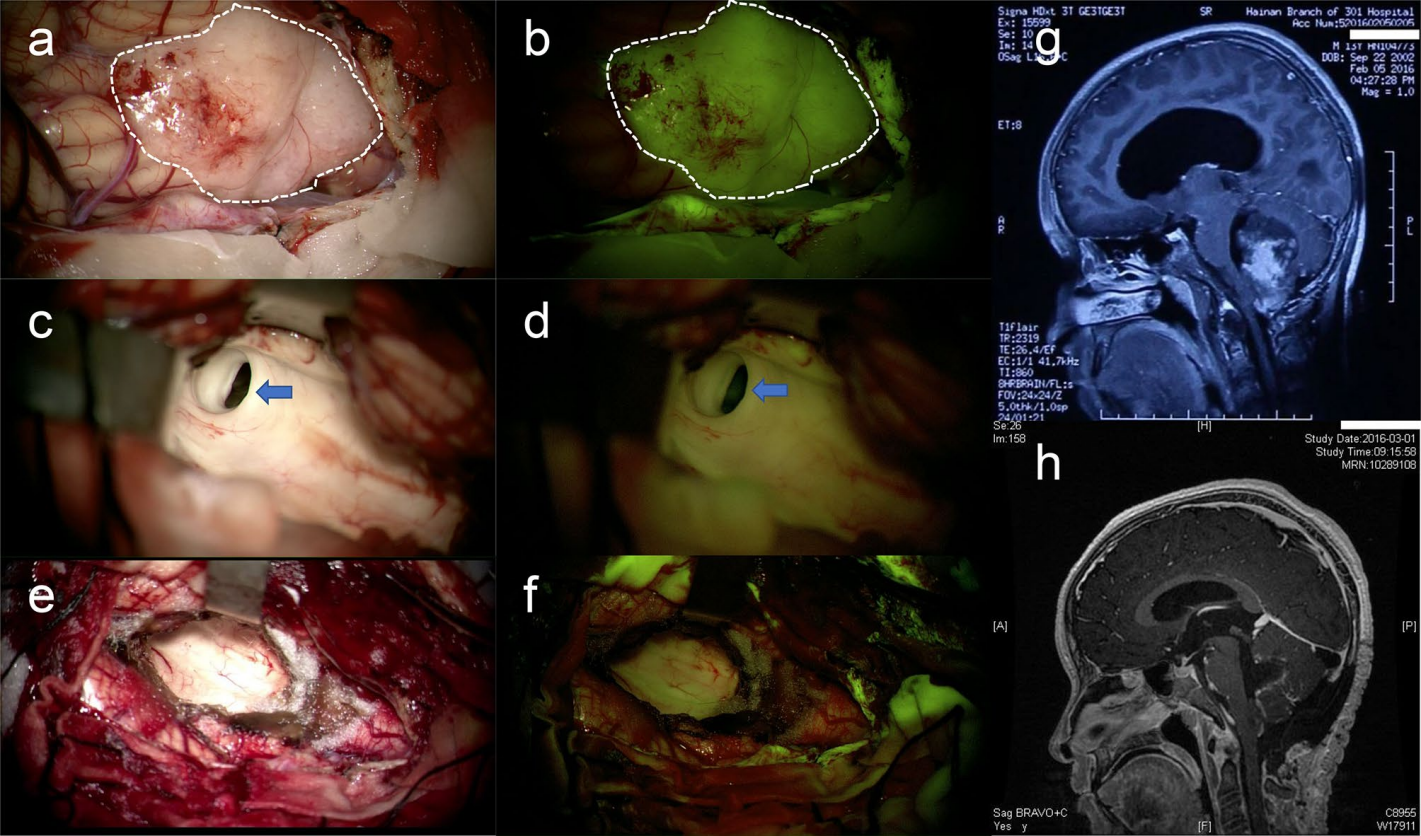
An 8-year-old boy underwent fluorescein sodium guided surgery for medulloblastoma of the cerebellar vermis. a The tumor (showed the white dashed frame) herniated from the cerebellomedullary fissure under the white light and (b) the yellow light. When the tumor was removed, the opening of the midbrain aqueduct (c, the white light mode; d, the yellow light mode) (blue arrow) and the fourth ventricle were revealed (e, the white light mode; f, the yellow light mode). g, h Sagittal magnetic resonance imaging shows complete removal of the tumor
